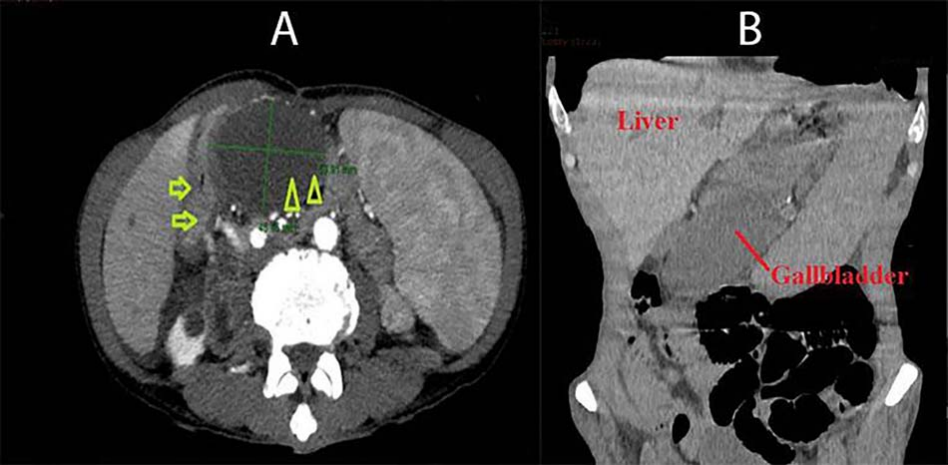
Abdominal CT images: A (axial view): duodenum (arrow) and the gallbladder located on the left of the duodenum and posterior to the stomach (arrow head); B (coronal view): the gallbladder was situated under the stomach and far from the gallbladder bed.
